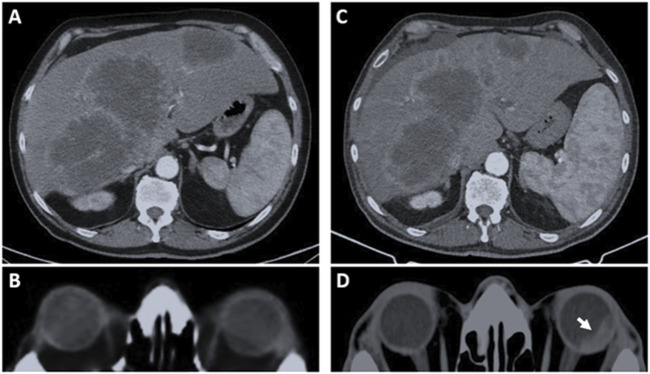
Selected computed tomography (CT) images before and after first-line therapy. Panels A and B refer to the baseline CT assessment (October 2024). (A) Multiple hypodense hepatic lesions with peripheral contrast enhancement are evident in both lobes. The largest lesion, measuring approximately 13 cm, is located in segments VII–VIIs. (B) The cranial-encephalic evaluation shows no evidence of choroidal involvement, and the patient reported no ocular symptoms at that time. (C) Post-treatment reassessment (March 2025) shows substantial stability of hepatic disease, but reveals a newly detected choroidal lesion in the left eye as indicated by the white arrow (D), with an estimated extent of approximately 18 mm.
